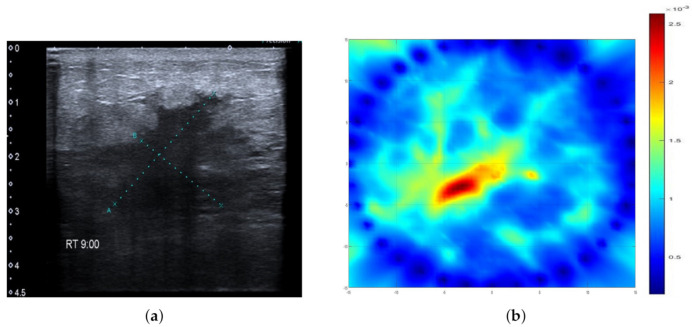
Comparison of SAFE lesion detection with clinical ultrasound: (a) ultrasound scan showing malignant lesion in the right breast and (b) SAFE scan showing the concordance with ultrasonography.
